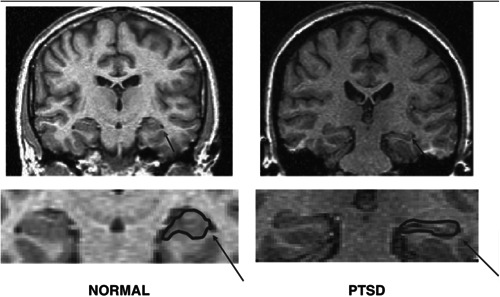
Reduced hippocampal volume in post‐traumatic stress disorder on a coronal functional magnetic resonance imaging (850 × 505). Propriety of James Douglas Bremner (2015). Traumatic stress from a multiple‐levels‐of‐analysis perspective. Developmental psychopathology, Second edition (pp. 656–676). doi:10.1002/9780470939390.ch16.
